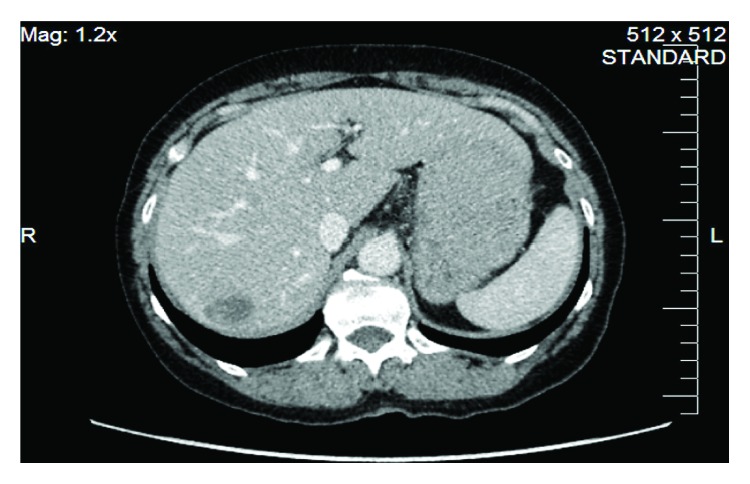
Abdominal CT showing 2.9 cm mass in liver segment 6/7.
